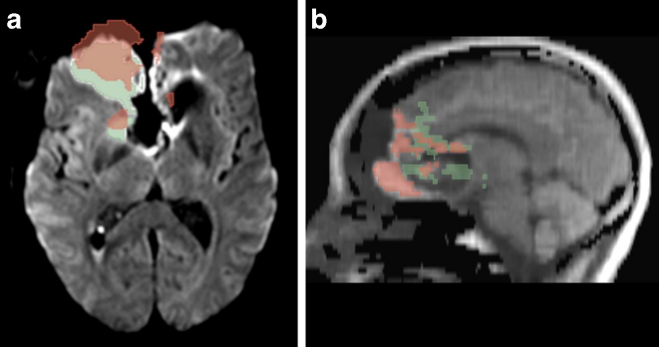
3D Slicer segmentation tool. Typical example of different segmentations of postoperative STEAM (*red*) and EPI (*green*) diffusion-weighted images with b = 1000 s/mm^2^. The segmentations are projected onto (a) an axial EPI DWI slice and b a reconstructed sagittal STEAM DWI slice of the same patient. The distortion of EPI DWI due to air artifacts in the frontal base of the skull becomes obvious
